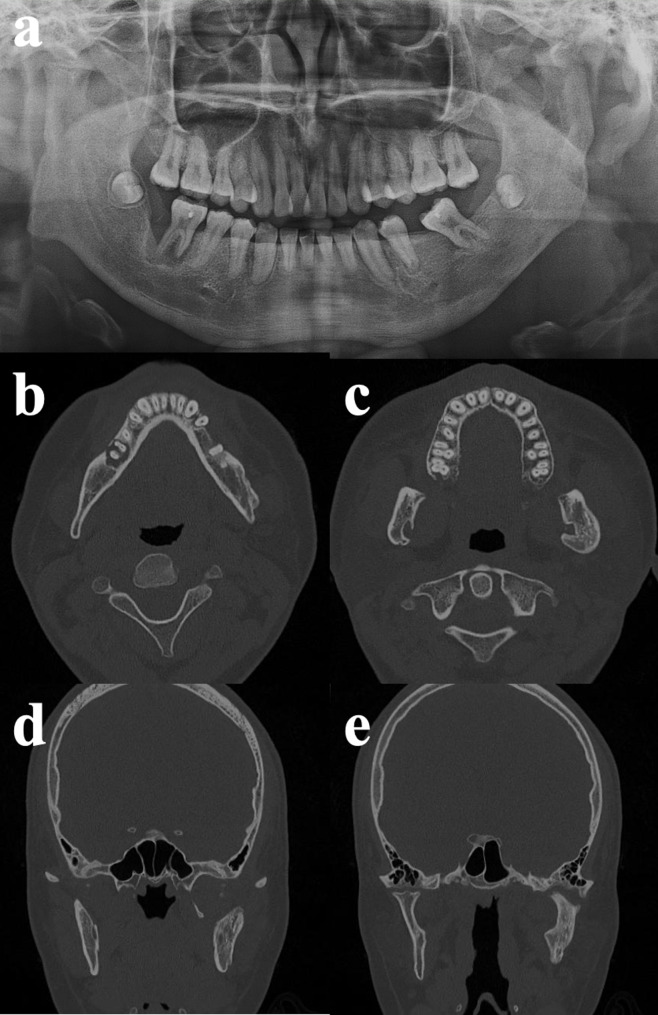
Nine-month follow-up after discharge. a Panoramic radiograph. b, c Axial CT images. d, e Coronal CT images
